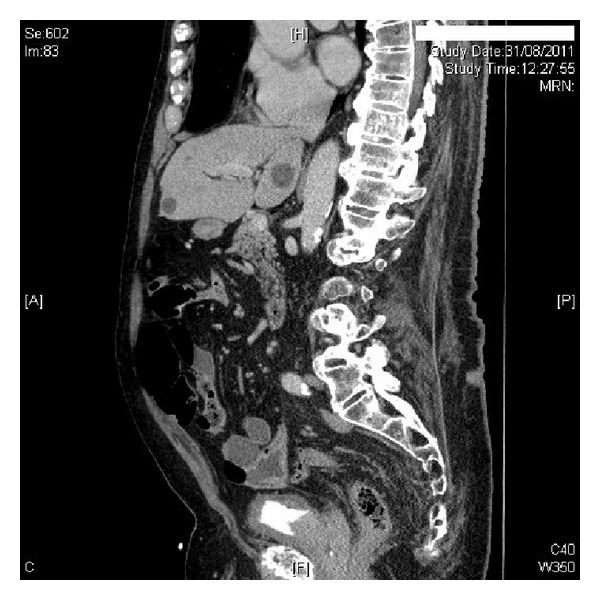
CT urography (sagittal view) revealed an irregular mass at the base of urinary bladder, which was likely to be an irregularly enlarged median lobe of prostate rather than intrinsic bladder neoplasm.
